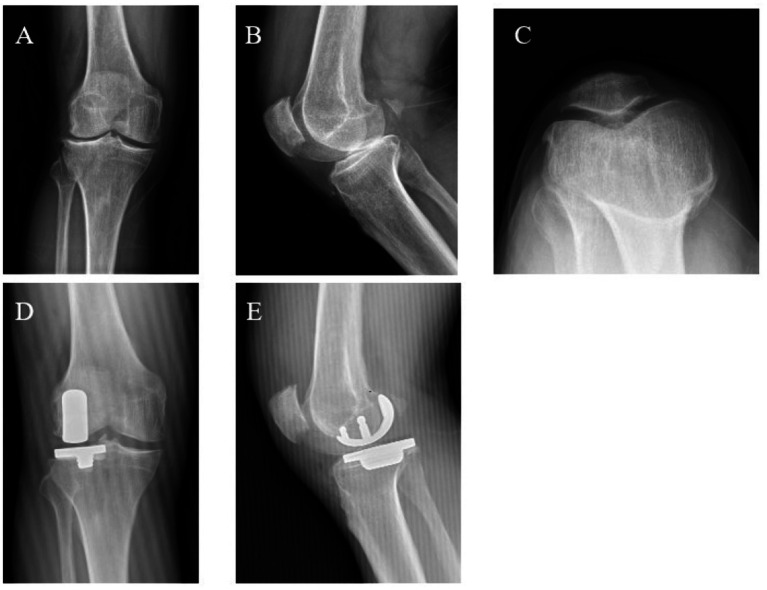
Representative radiographs of a patient treated with LUKA. (A–C) Preoperative anteroposterior (AP), lateral, and skyline (axial) views showing LCOA with mild patellofemoral degeneration. (D,E) Postoperative AP and lateral views demonstrating no obvious malpositioning on routine radiographs.
